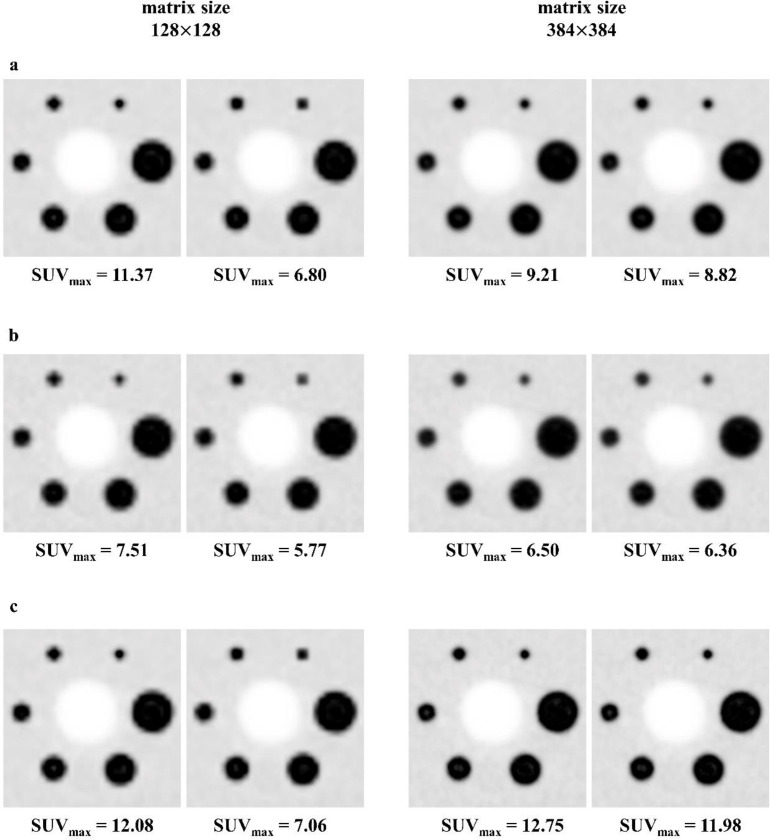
PET images reconstructed at different reconstruction center position. Matrix sizes are 128×128 and 384×384 at a TBR of 8. The images reconstructed with OSEM + PSF + Filter (a), OSEM + Filter (without PSF) (b), OSEM + PSF + no Filter (c). The values at the bottom of the images are the maximum and minimum SUV_max_ of the 10-mm hot sphere for each reconstruction settings
